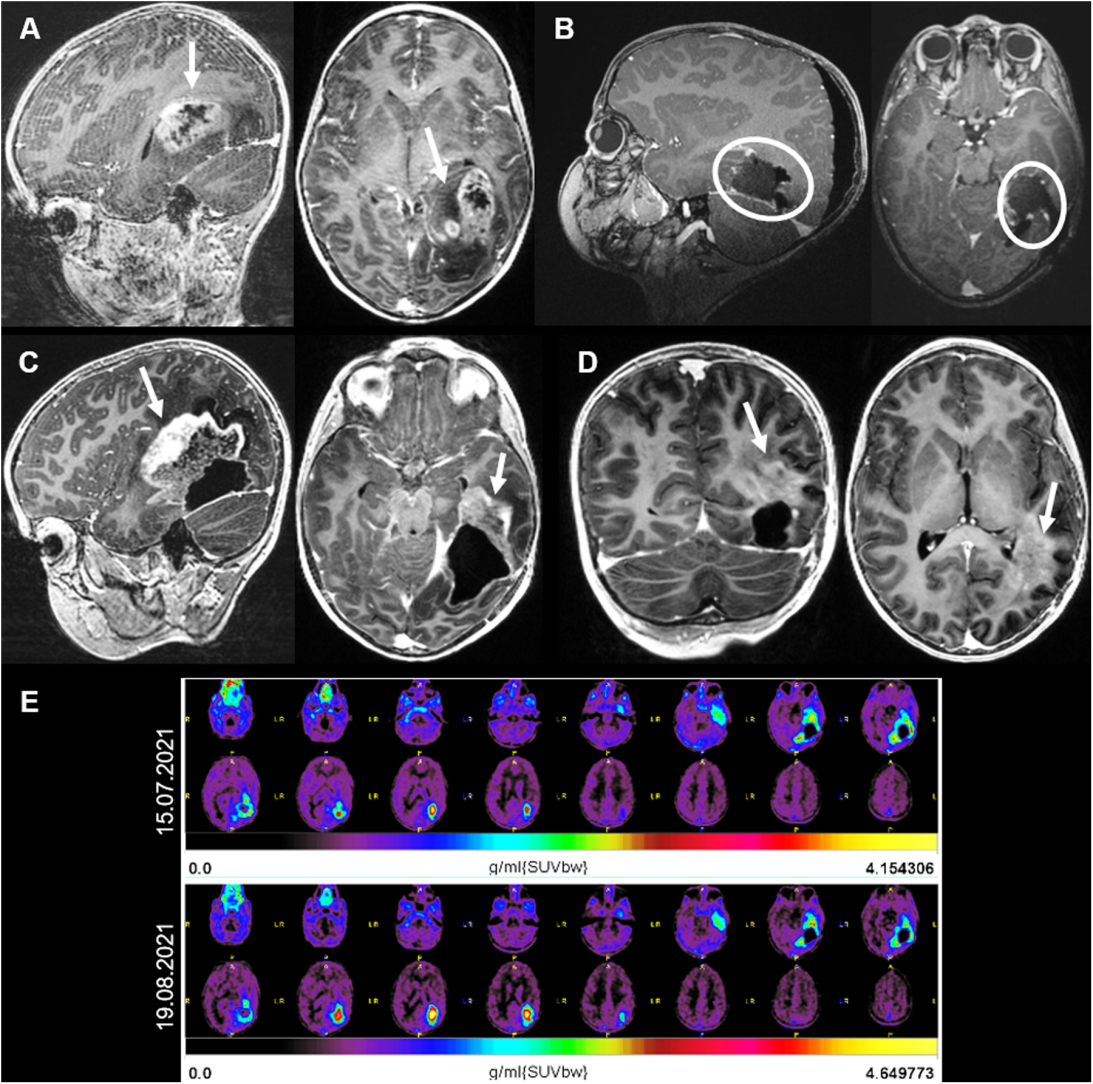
(A) Case 2 sagittal, axial and coronal MRI T1 sequences with contrast. (A) MRI showing an intra-axial tumor on the left fusiform gyrus with contrast enhancement and central necrosis. (B) Postoperative MRI showing a gross total resection. (C) Three months after surgery and adjuvant radiotherapy, radio-necrosis reaction (arrows) was superiorly and anteriorly observed. (D) One month later, regression of the radio-necrosis but evidence of tumor progression on the left tapetum (arrow). (E)
